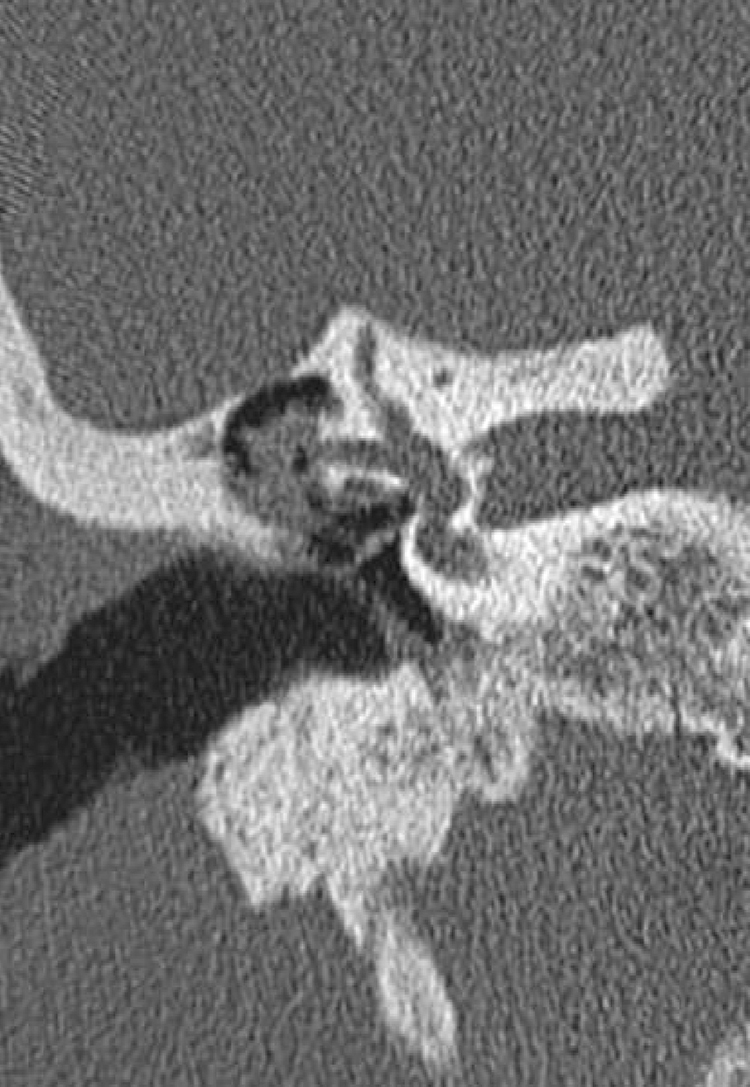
LF (lateral semicircular canal) in a CT scan.
